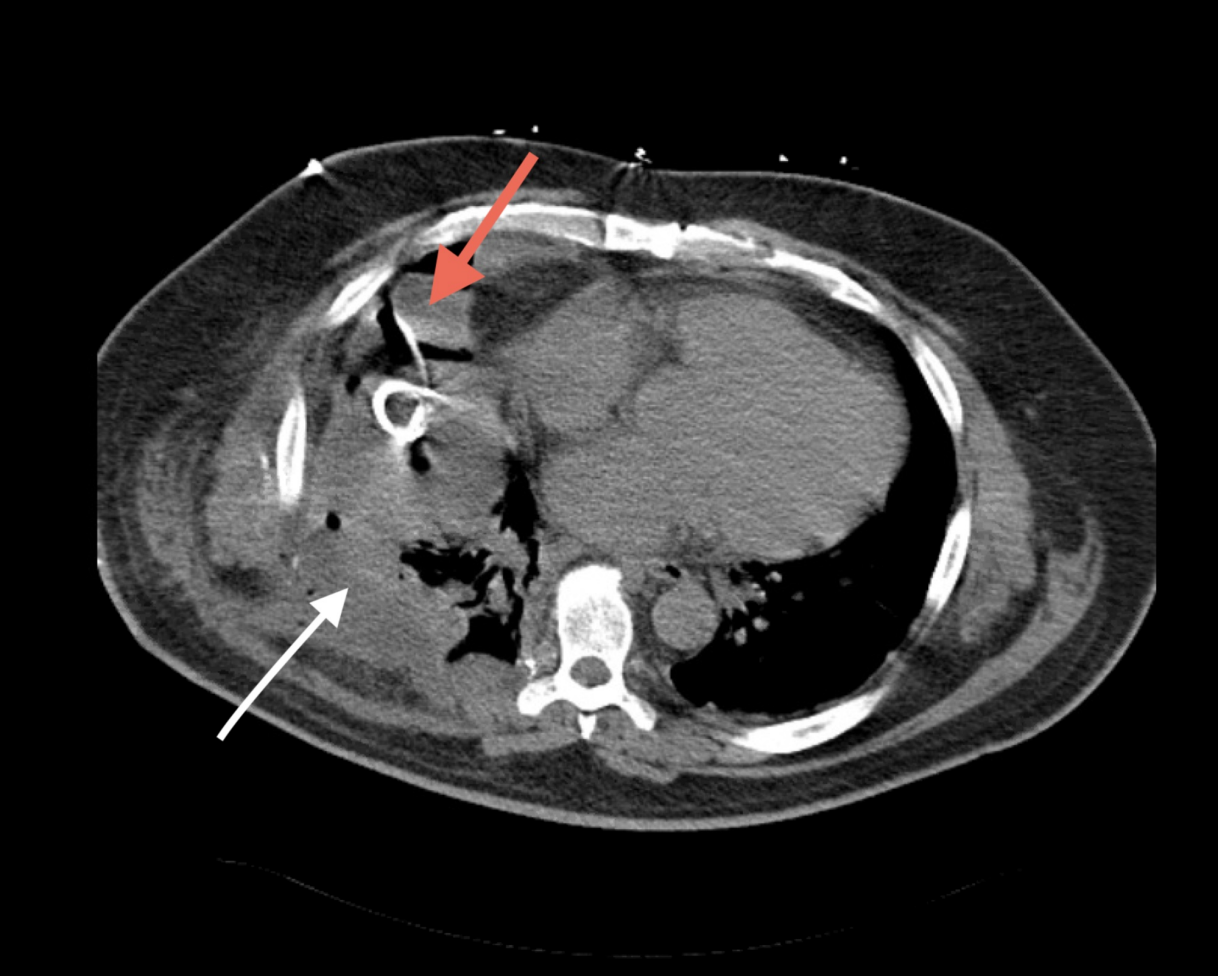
CT of the chest with a dominant fluid collection in the anterior aspect of the right chest (red arrow). There is also a loculated collection in the posterior aspect, with multiple septae (white arrow).
